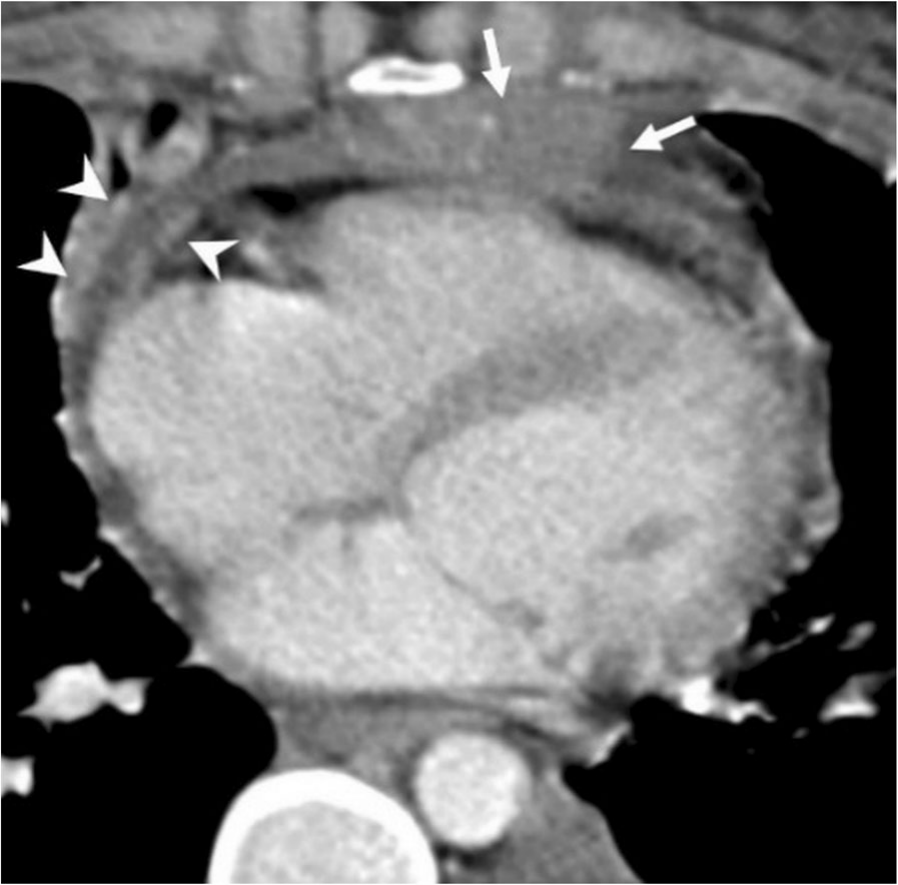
A 26-year-old woman with pathologically proven mediastinal lymphoma. Axial contrast-enhanced CT image demonstrates the inferior aspect of the mediastinal mass (arrows) and pericardial nodular areas of contrast enhancement and thickening suggestive of pericardial involvement (arrowheads)
